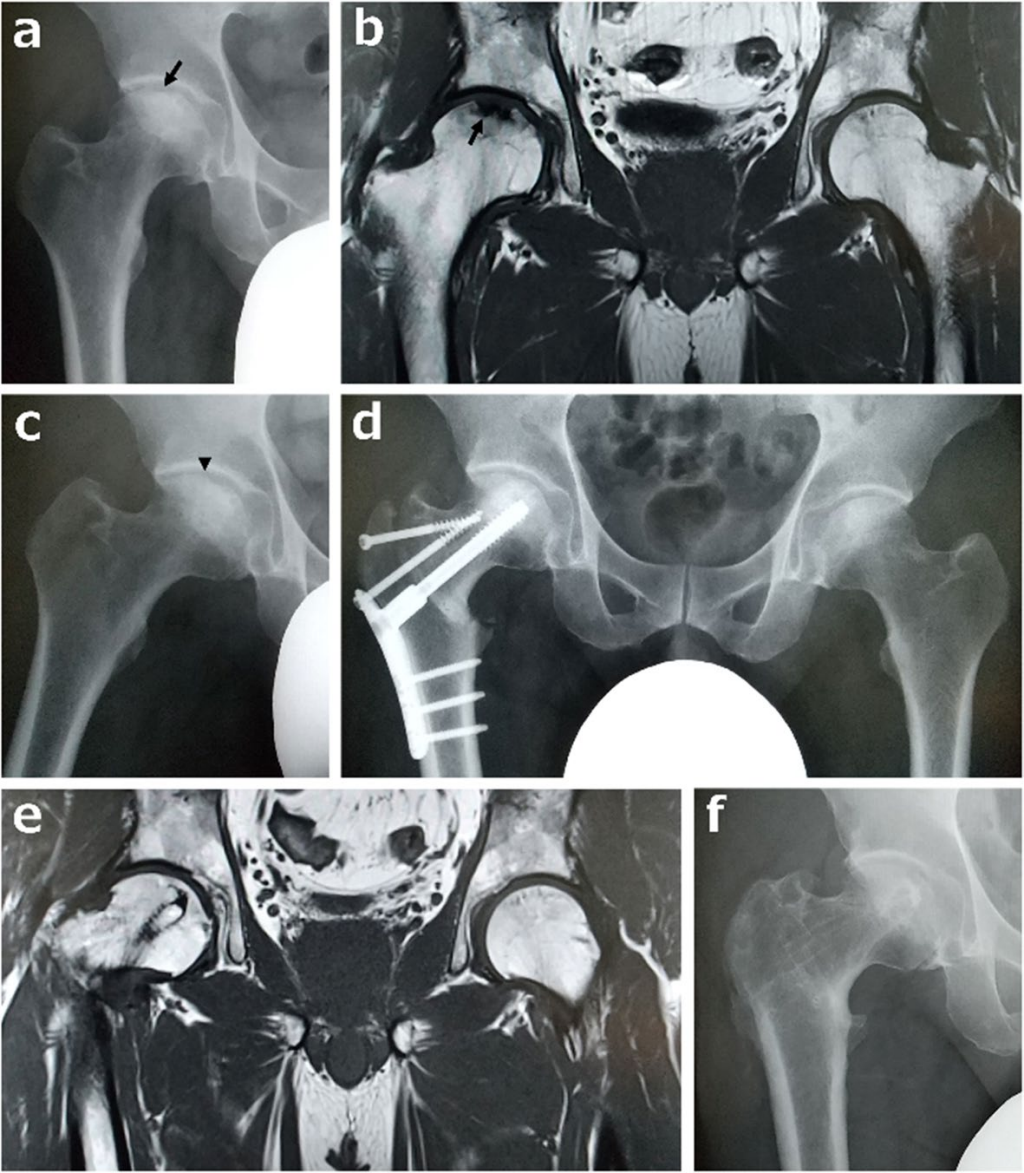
Case 4 (a 49-year-old man). a An anteroposterior (AP) radiograph of the right hip at the time of onset shows the collapse of the weight-bearing region of the right femoral head (arrow). b T1-weighted magnetic resonance image (MRI) shows a low-signal area and low-intensity band (arrow) in the weight-bearing region of the right femoral head. c Preoperative AP radiograph of the right hip in maximum abduction. The arrowhead indicates the lateral end of the fractured area. d Six months after onset, inter-trochanteric curved varus osteotomy was performed. e T1-weighted MRI 2 years postoperatively. The low-signal area that was present preoperatively disappeared. f An AP radiograph taken 6 years postoperatively showed fusion of the osteotomy site and non-progression of the femoral head collapse
